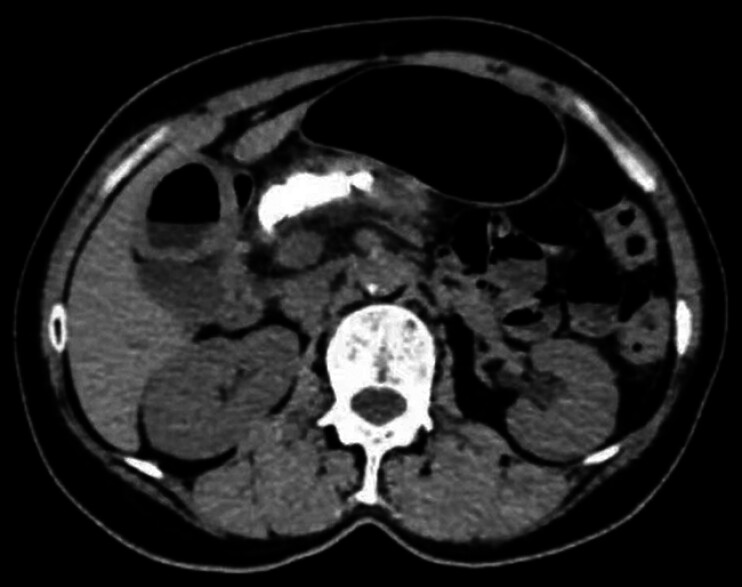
Computed tomography (CT) image showed pancreatic cancer, stenosis of the common bile duct and pancreatic duct, with the stenosis impinging on the horizontal part of the duodenum, and obstruction of the superior mesenteric artery and vein.
